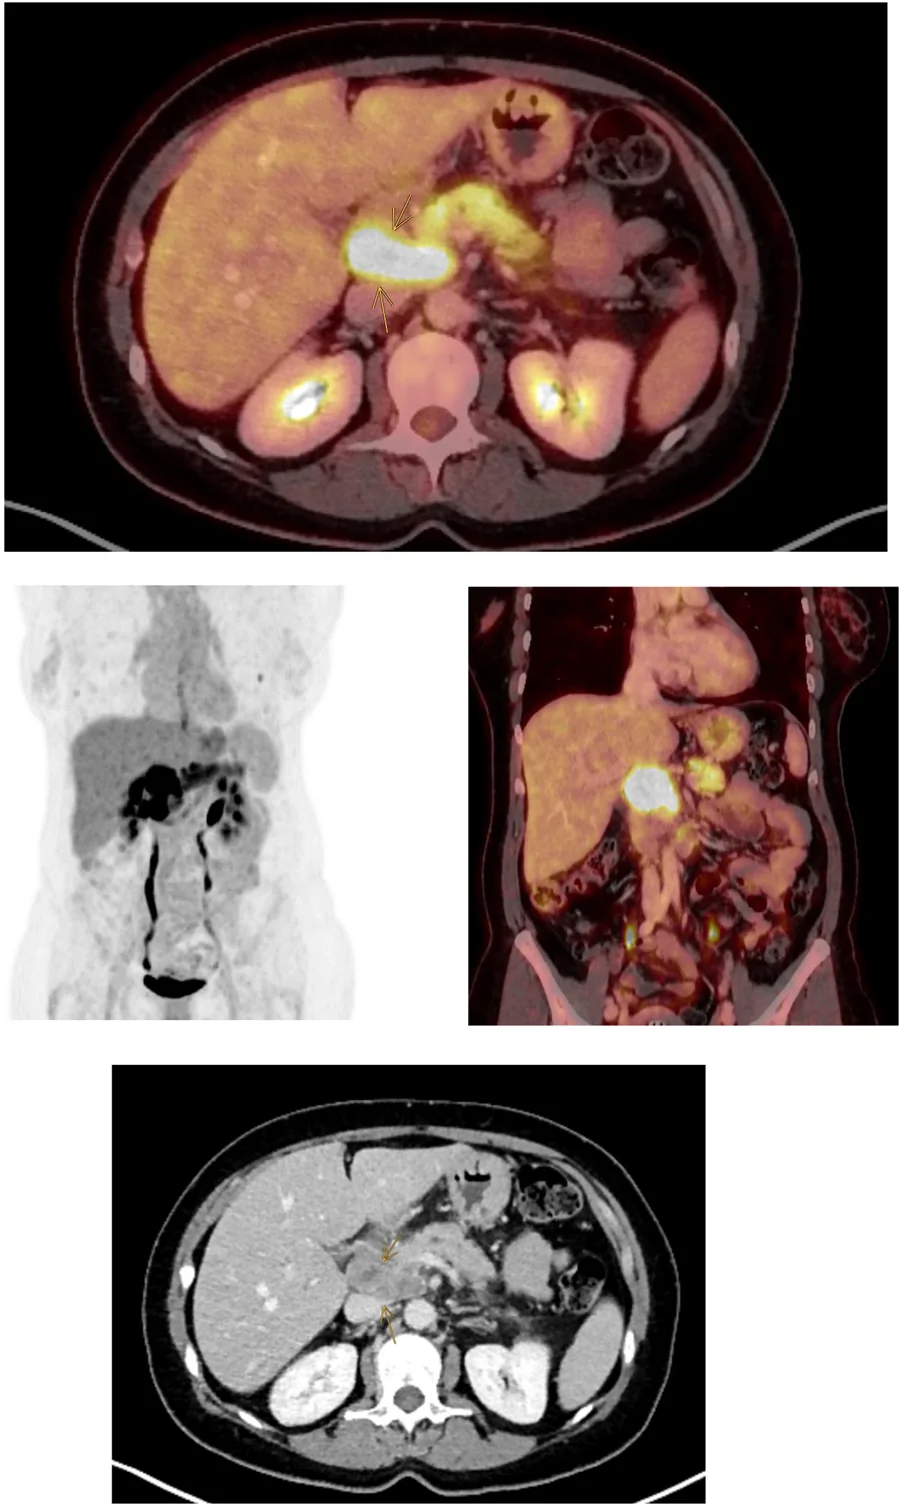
FDG-PET-CT. Large metabolically active mass measuring 2.3 × 4.8 cm between the portal vein and inferior vena cava.
